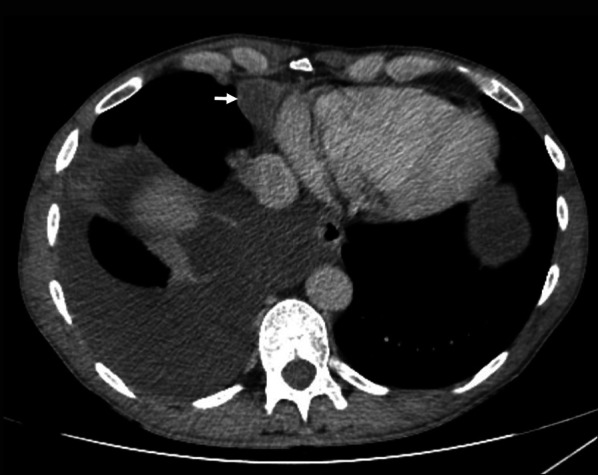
CT image of a patient with malignant peritoneal mesothelioma and concurrent pleural disease shows pleural based nodules (arrow) and pleural effusion
